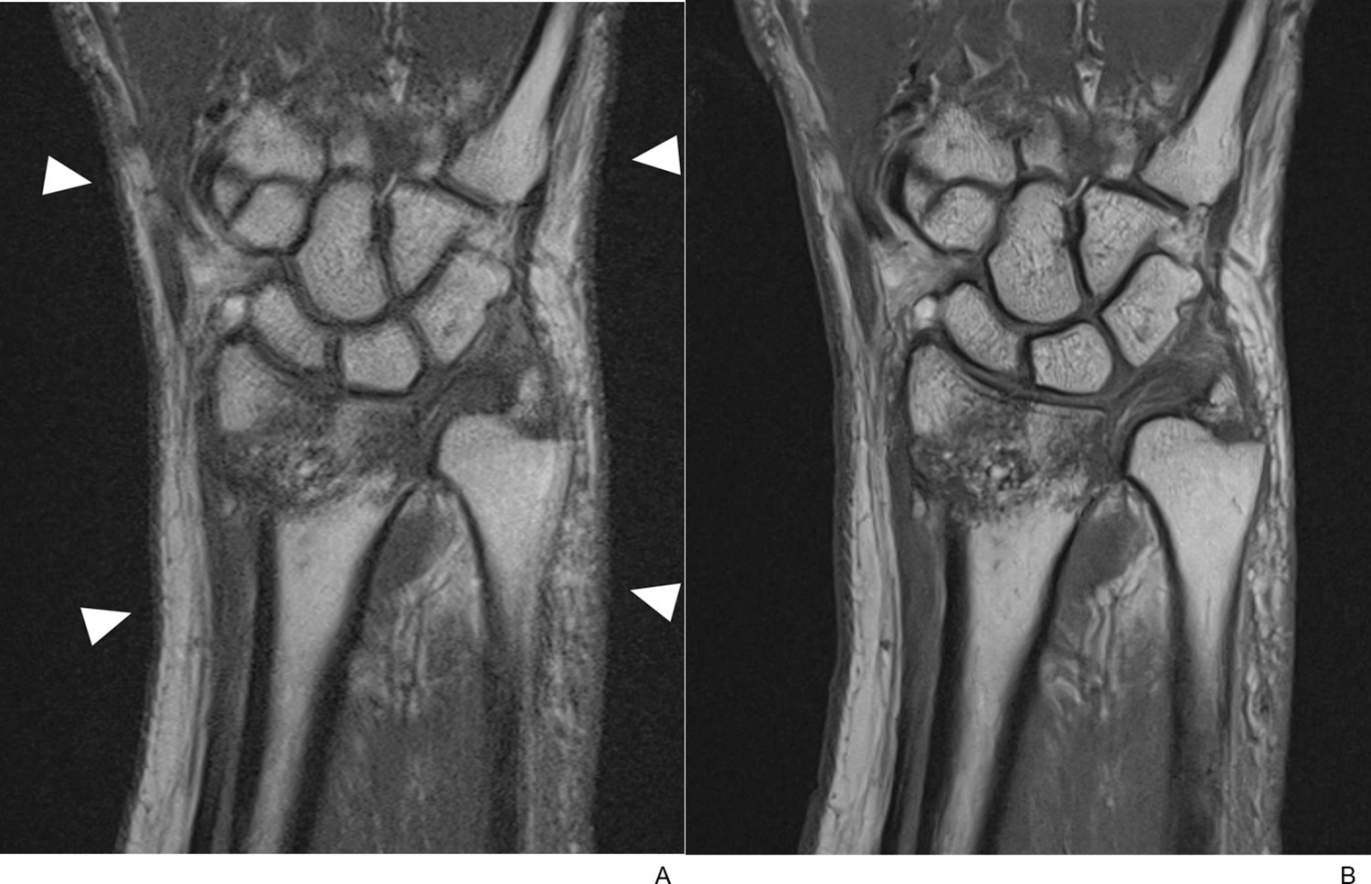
Motion artifacts. (A) Coronal T1-weighted TSE_S_ image of a 70-year-old female patient revealed severe margin blurring of the bone and joint by motion artifact (arrowheads). (B) In contrast, sharp-edged anatomic details were noted without motion artifacts in the corresponding TSE_DL_ image.
